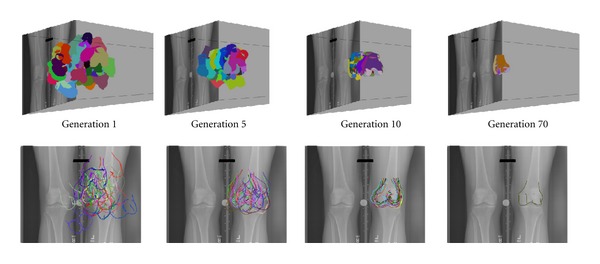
Multiple evolutionary generations in the CMA-ES optimization approach show a steady march toward a single best alignment (top row), driven by a cost function that incorporates evaluation of agreement between a model silhouette projected onto the radiographic image plane and the bone edge detected from the actual radiograph (bottom row). Each CMA-ES generation includes 70 candidate spatial alignments, with those having the lowest cost function evaluation being used to drive subsequent generations.
